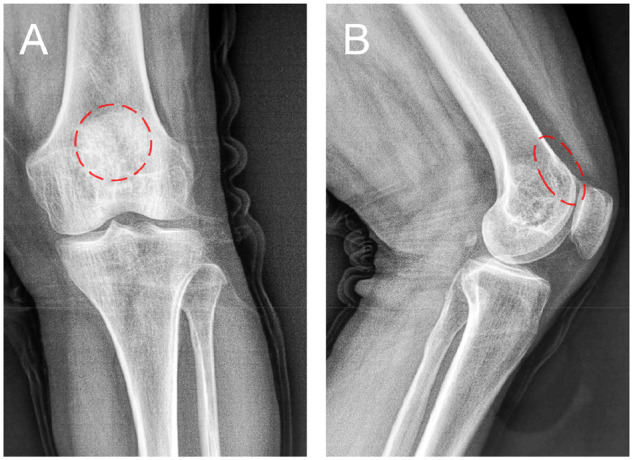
Frontal (A) and lateral (B) views of plain radiography at 24 months after the operation (the surgical site is noted by dashed circle in each picture).
